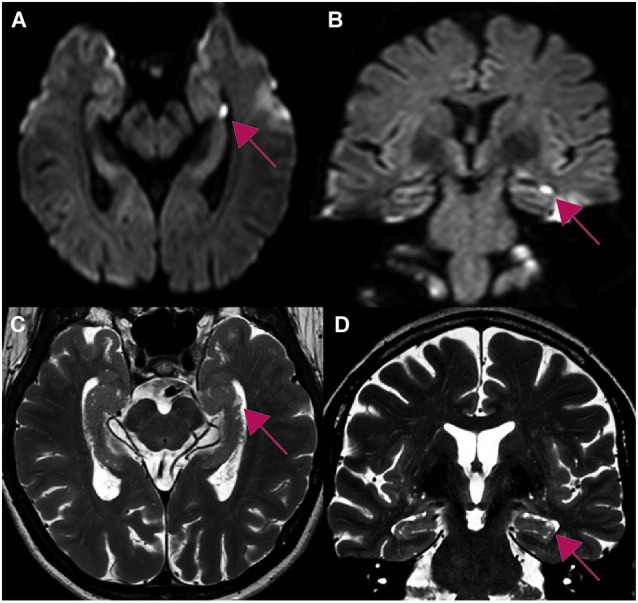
Whole brain 3T MR-imaging of one patient showing a focal lesion in the head of the left hippocampus in CA1 (red arrow) after an emotional stressful event. The lesion in the diffusion-weighted imaging (A,B) correlates with the lesion in T2-weighted sequences (C,D). Table 1: Study 2: demographic data, precipitating events and distribution of hippocampal lesions. Ant, anterior; post, posterior.
